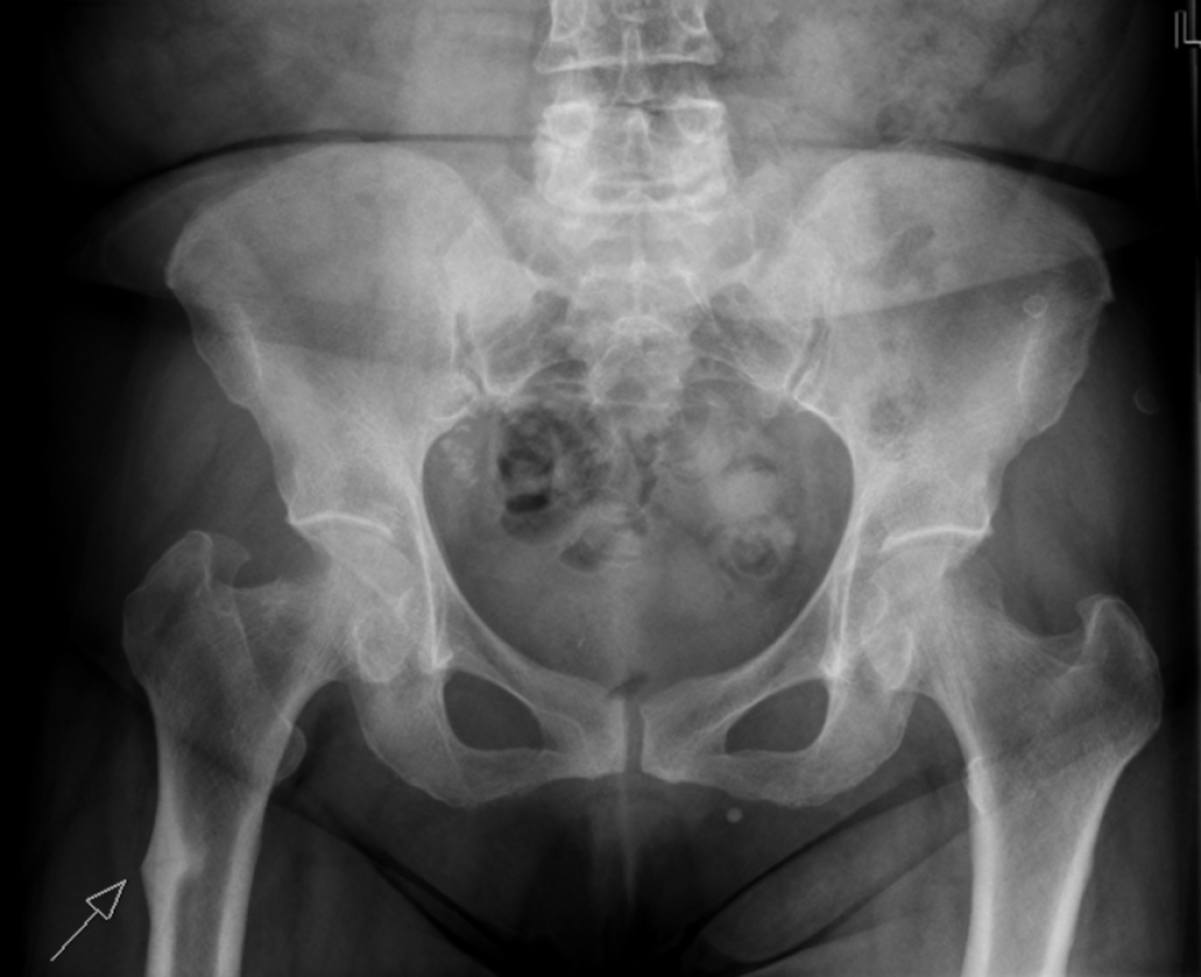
Pelvic X-ray shows bilateral atypical femoral fractures
